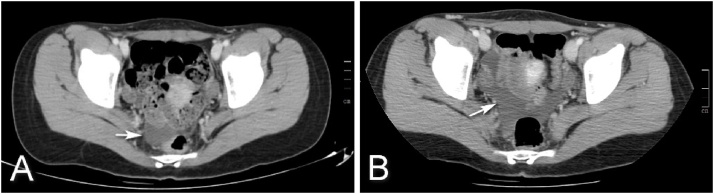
Computed tomography image on admission (image A) and after 24 h surveillance (image B) with an increase in the amount of peritoneal fluid (arrow).
